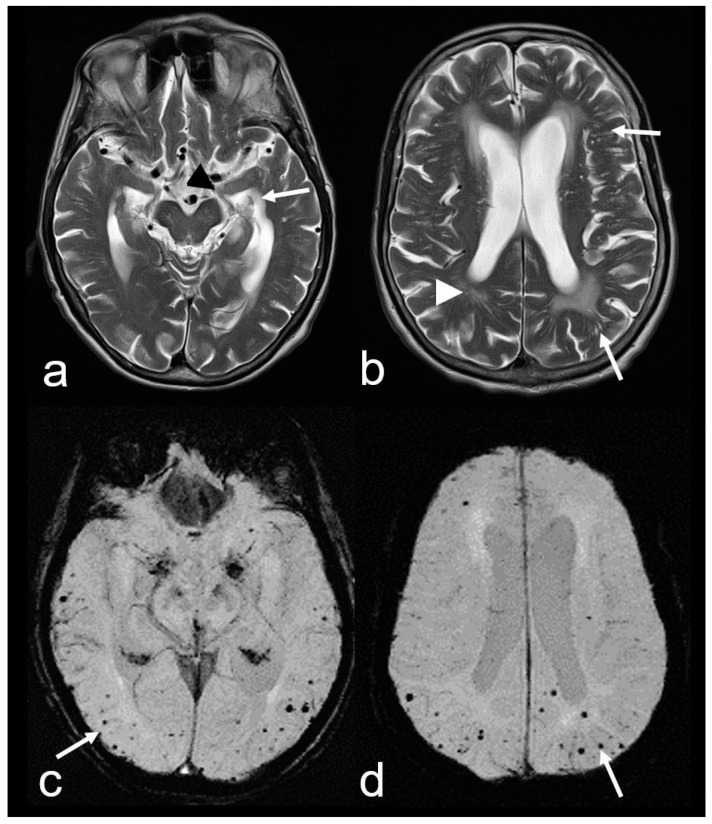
Cerebral amyloid angiopathy (CAA) and Alzheimer’s disease (AD) in an 82-year-old woman with progressive cognitive decline and short-term memory disturbance. Axial T2-weighted images (WI) (a,b) showing distinct temporal mesial atrophy ((a), arrowhead), enlarged temporal horns ((a), arrow), vascular leukoencephalopathy ((b), arrowhead) and enlarged perivascular spaces (PVS; (b), arrow). Susceptibility-weighted imaging (SWI) ax. (c,d) disclosing multiple cortical and subcortical microbleeds (MB) (arrow), especially temporal and parietal; MRI 1.5 T Siemens AREA.
